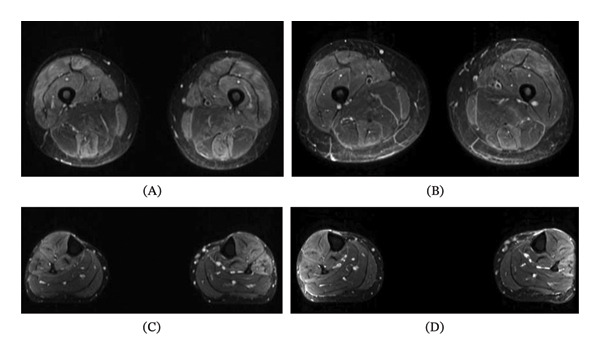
MRI (STIR sequence) findings of the muscles of the lower extremities. (A) MRI of thighs in October 2022 showing edema of the quadriceps femoris, gracilis, and semitendinosus muscles. (B) MRI of thighs in May 2023 showing slight resolution of the edema of the quadriceps femoris, gracilis, and semitendinosus muscles, but no muscle atrophy. (C) MRI of shanks in October 2022 showing edema in the tibialis anterior and peroneus muscles. (D) MRI of shanks in May 2023 showing edema in the tibialis anterior muscles and peroneus muscles with no muscle atrophy.
